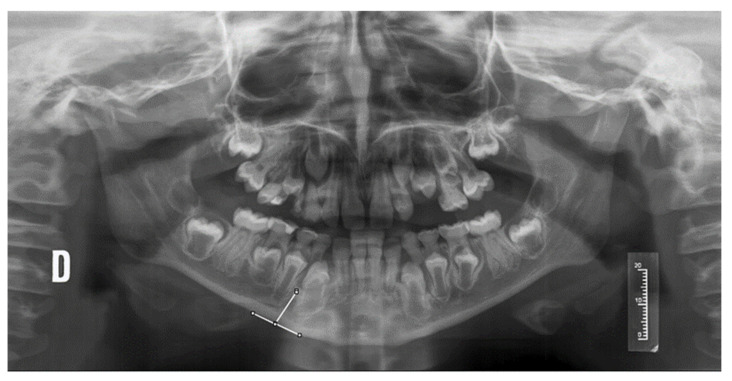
Measurement of the MCW index in an orthopantomography of a patient with OI. D: right side of the patient.
